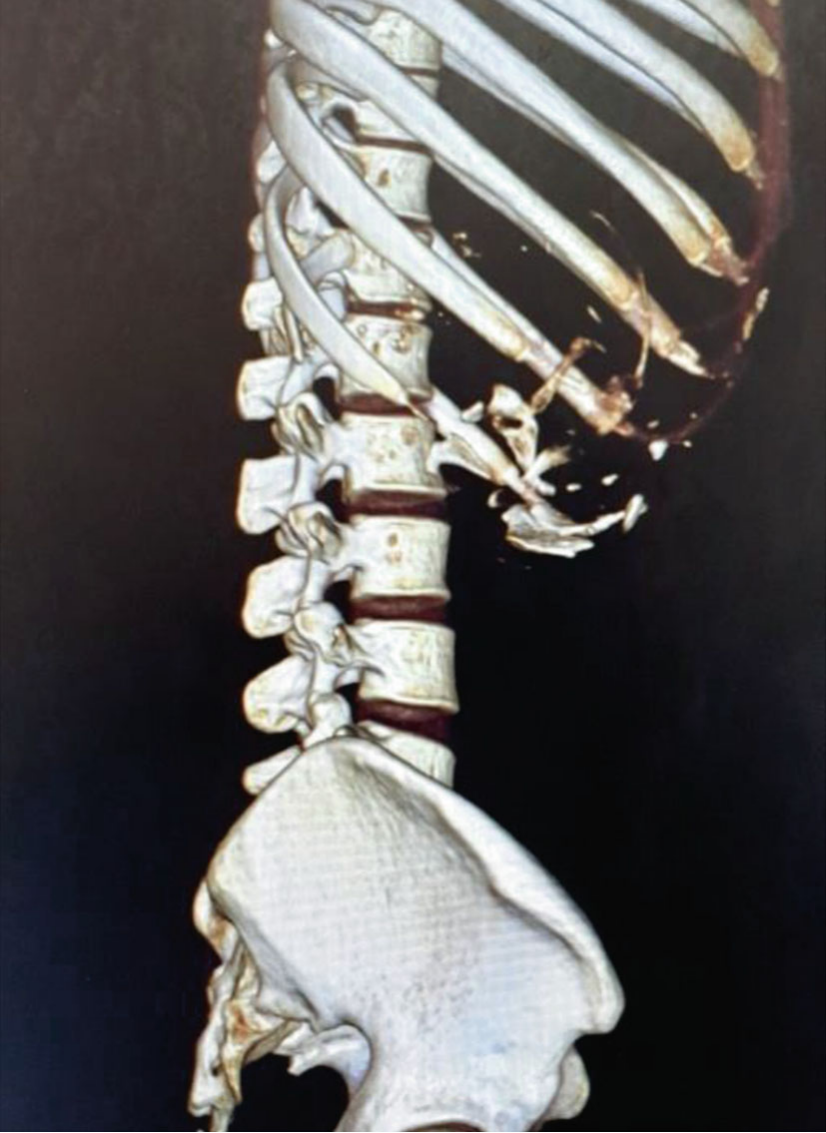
Three-dimensional reconstruction CT image showing bony outlines of fetus in fetu.
